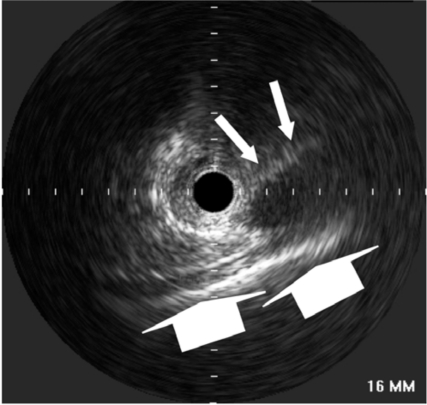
Intravascular ultrasound image obtained from the cardiac venous site. Please note the visibility of the pericardium (big arrows) and the coronary artery parallel to the vein (small arrows), enabling the orientation of TransAccess® catheter system.
